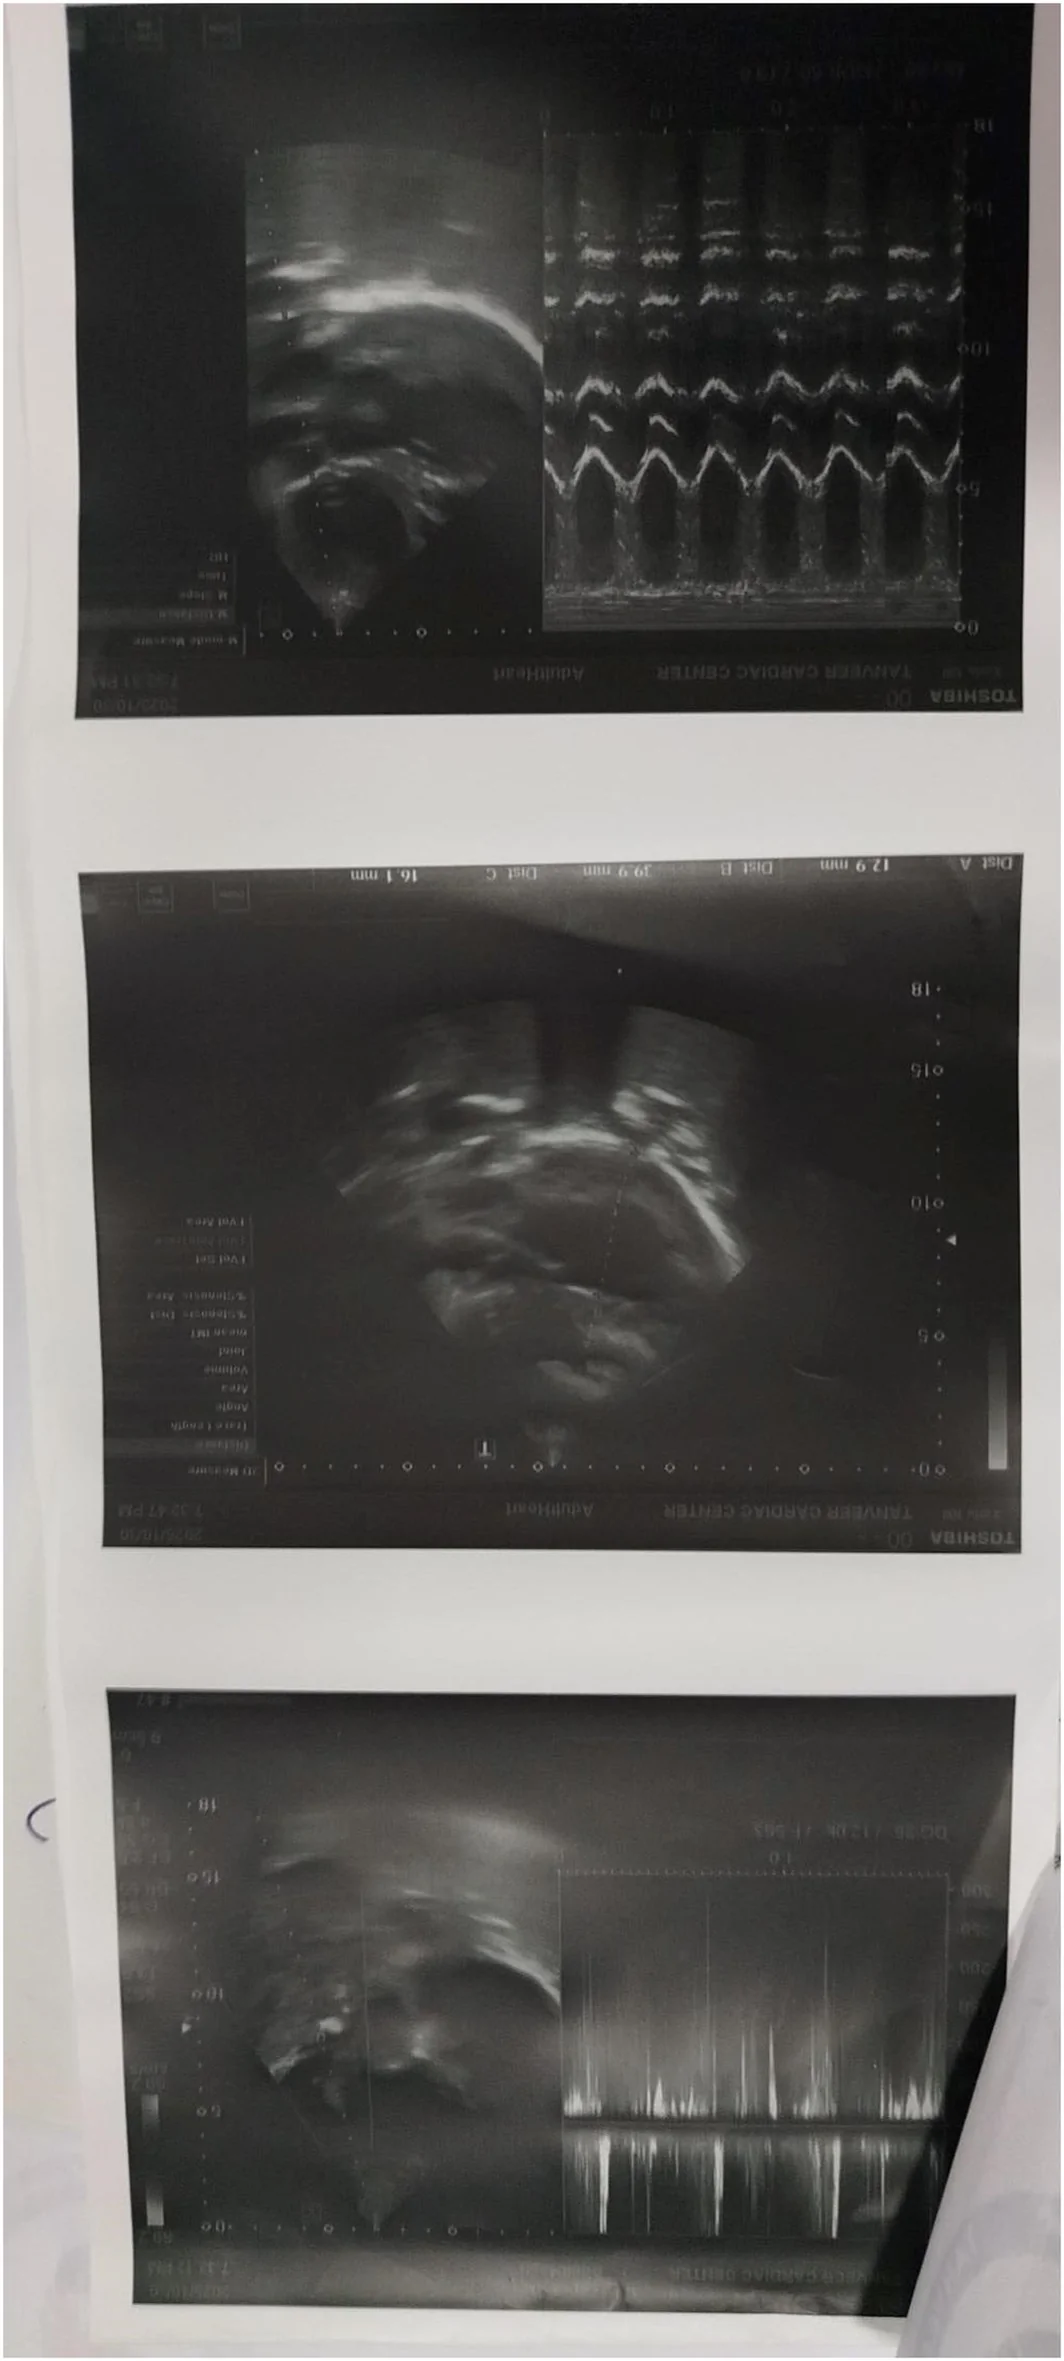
Two‐dimensional (apical four‐chamber view) and Doppler transthoracic echocardiographic images demonstrating right ventricular enlargement, Doppler‐estimated elevated pulmonary artery pressures, and significant pulmonary and aortic valvular regurgitation.
